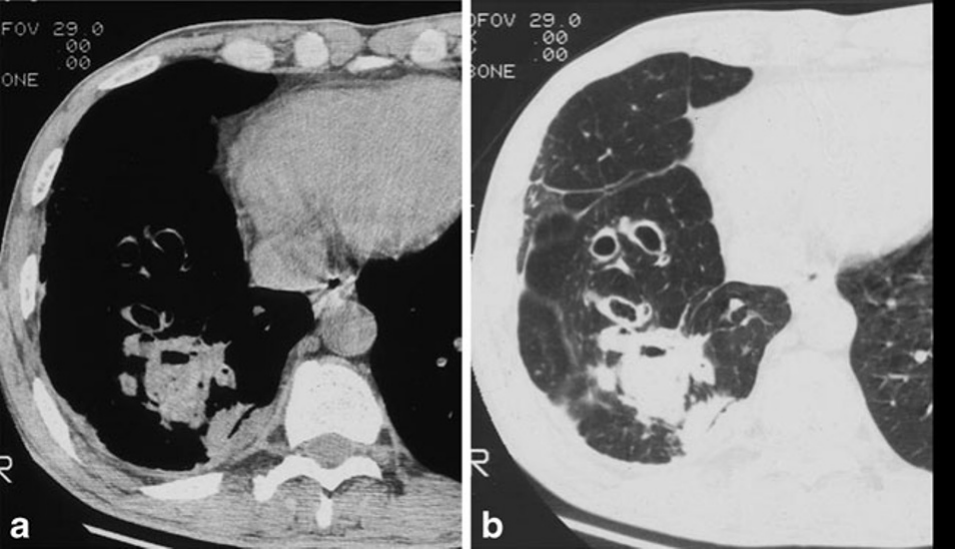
Bronchiectatic form of actinomycosis in a 44-year-old man with underlying bronchiectasis. Chest CT scan displayed on the mediastinal (a) and lung (b) window setting shows bronchial dilatation with wall thickening and focal consolidation. Note the adjacent pleural thickening in the right lower lobe
